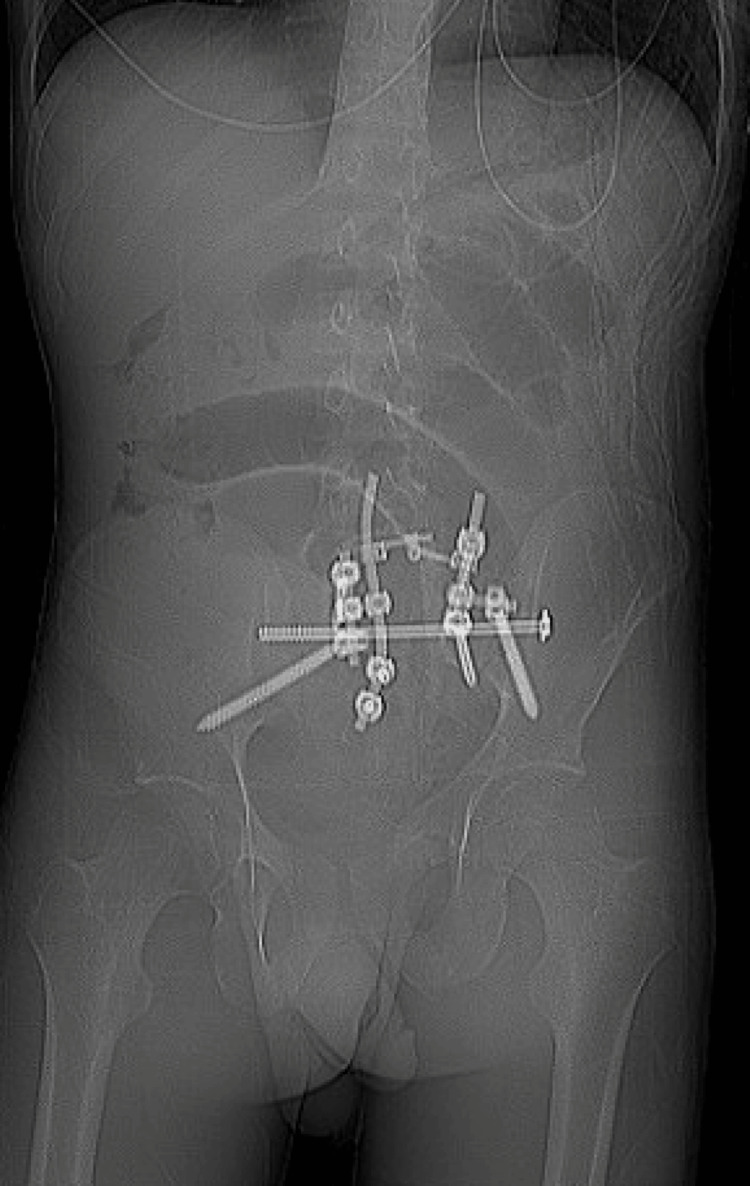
Follow-Up Post-operative X-ray Follow-up anteroposterior X-ray demonstrating hardware placement and bony fusion
